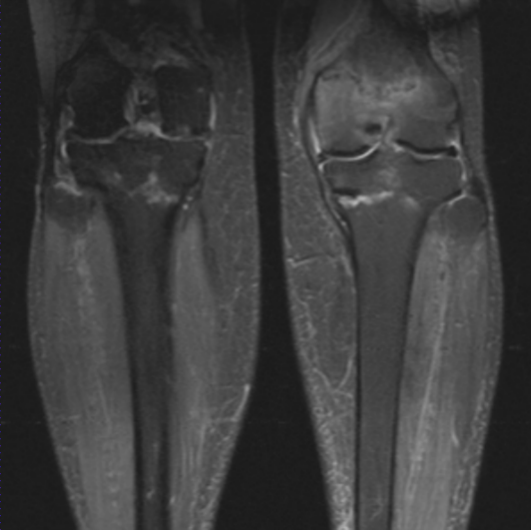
Magnetic resonance imaging coronal short inversion time inversion recovery images show a linear signal change in both the proximal tibia and left distal femur with surrounding reactive change.
